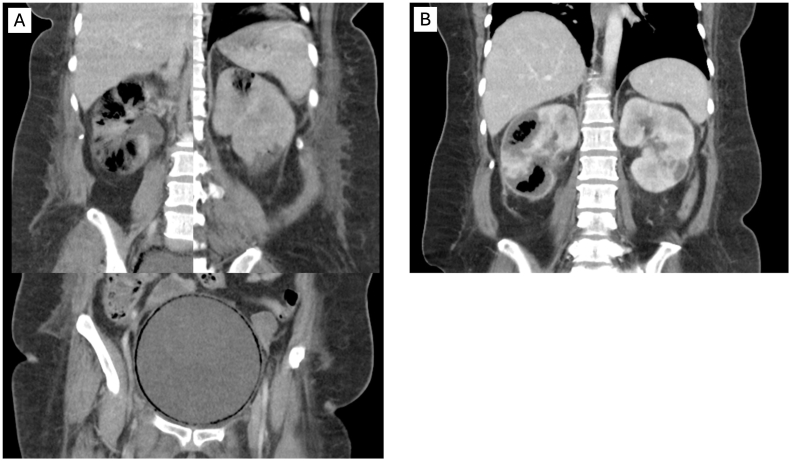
Imaging; CT abdomen pelvis. 1a)Bilateral Imaging CT abdomen pelvis on admission. 1b) CT abdomen and pelvis carried out 3 weeks after admission, after right percutaneous nephrostomy tube was accidently dislodged.
